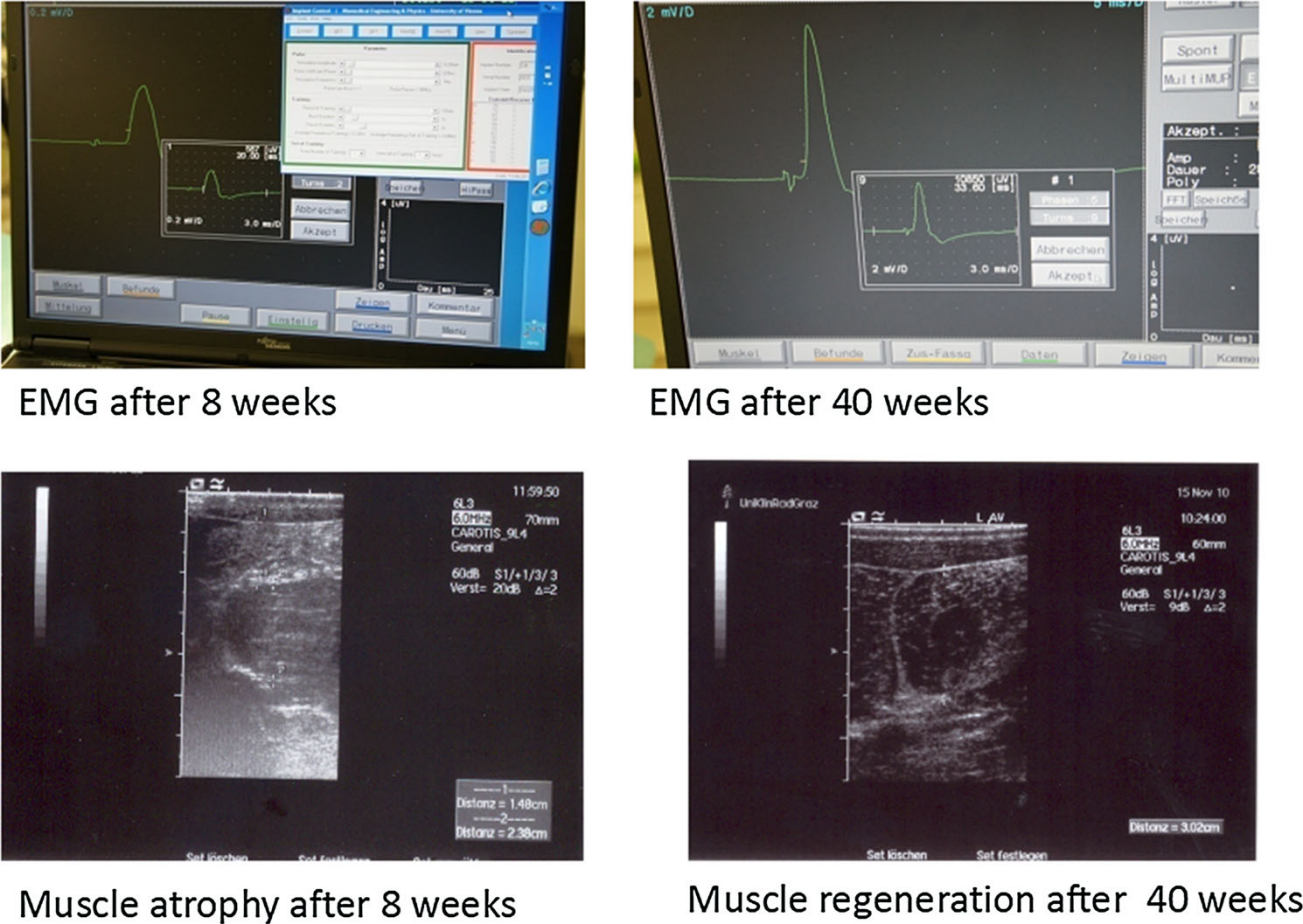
EMG and ultrasound measurement of the muscle
